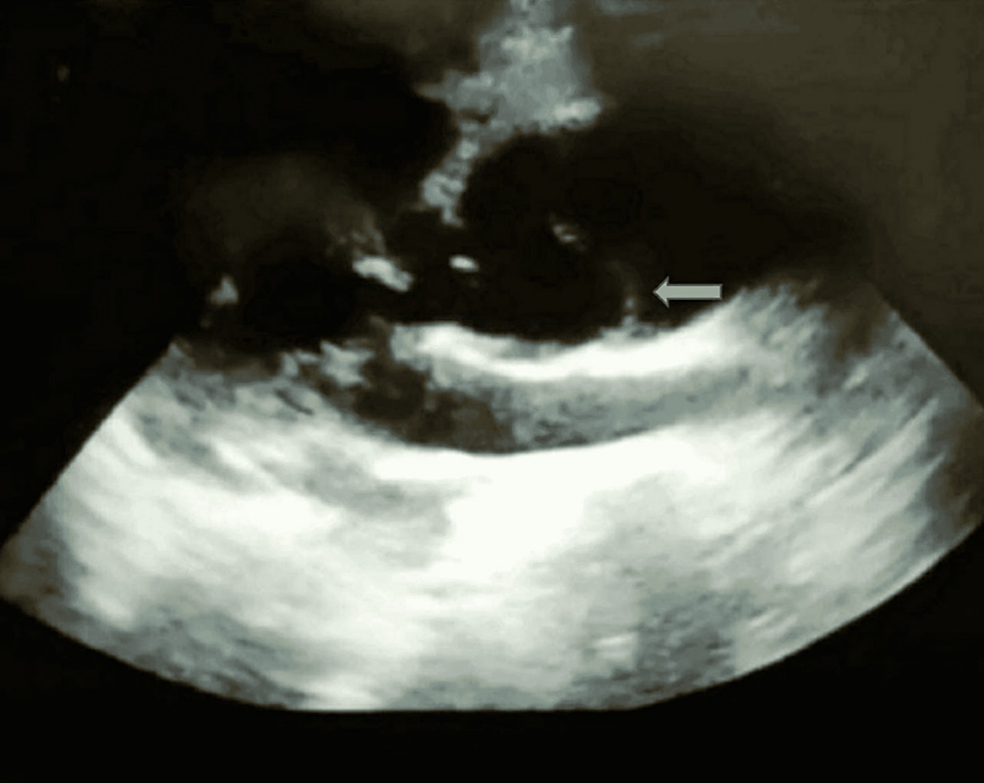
Intimal flap seen on parasternal long-axis view (arrow) (point-of-care ultrasound)
